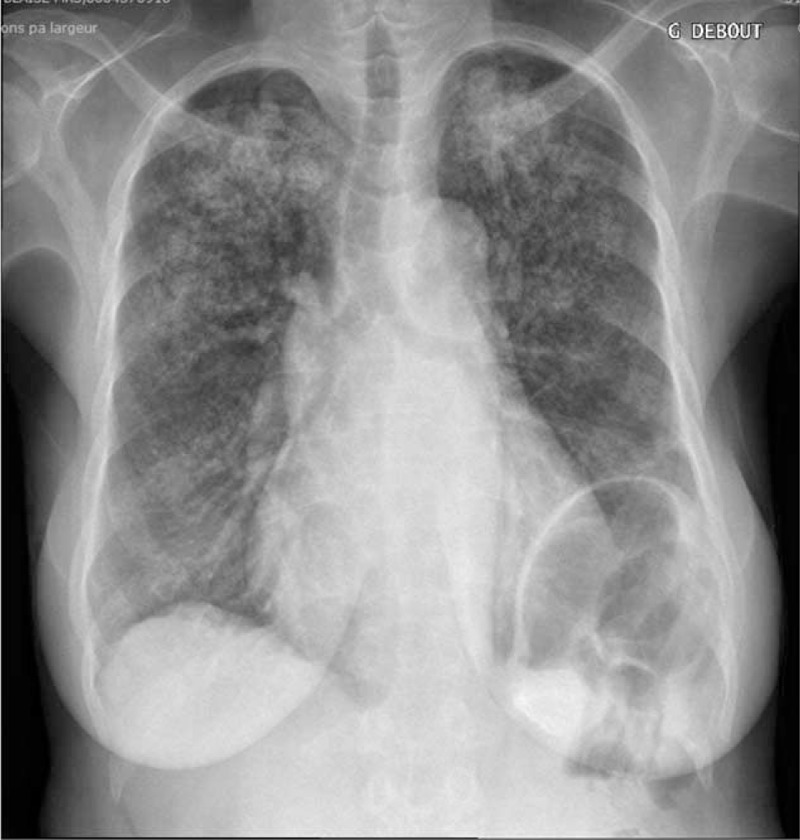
Chest x-ray at first clinical evaluation, initial chest x-ray showing diffuse calcifications, predominantly in the upper part of the lungs.
